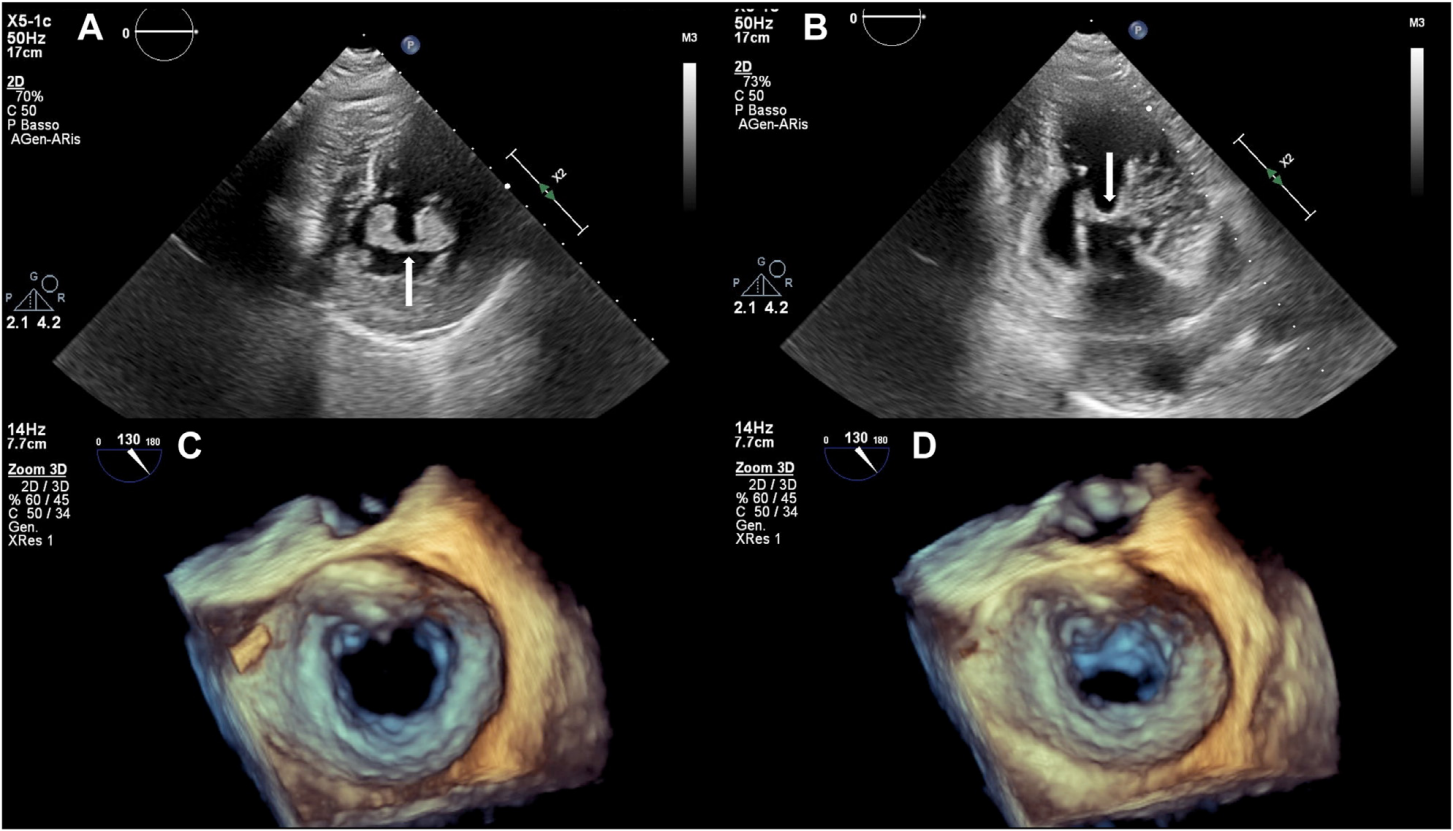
Two-dimensional TTE, parasternal short-axis (A) and apical 2-chamber (B) diastolic views demonstrate the anomalous fibrous bands (*arrows*) connecting the 2 hypertrophic papillary muscles. Midesophageal three-dimensional TEE, volume-rendered en face diastolic views of the MV from the left atrial (C) and LV (D) perspective demonstrate the normal opening of the leaflets and the narrowed subvalvular orifice.
